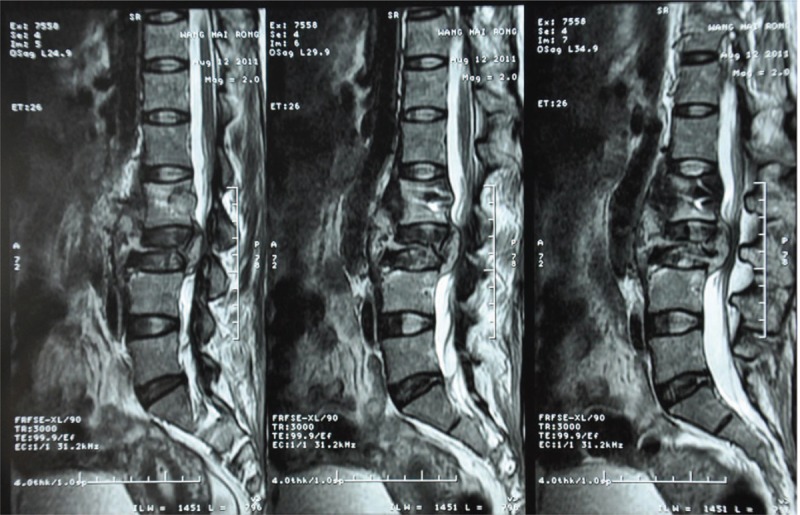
Preoperative MRI images demonstrated a cystic mass with solid content depressing the spinal cord. MRI = magnetic resonance imaging.
